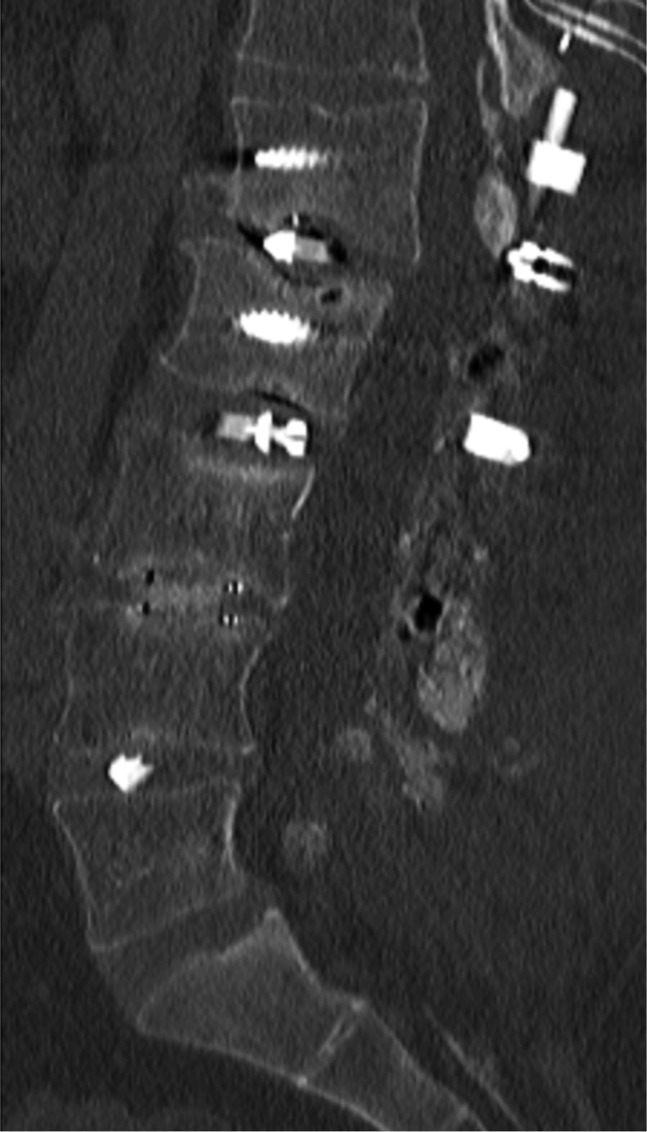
Sagittal CT lumbar spine. Demonstrating no evidence of bony incorporation across the disk space or posterior elements L1-L2 and L2-L3 and incomplete osseous incorporation L3-L4.
